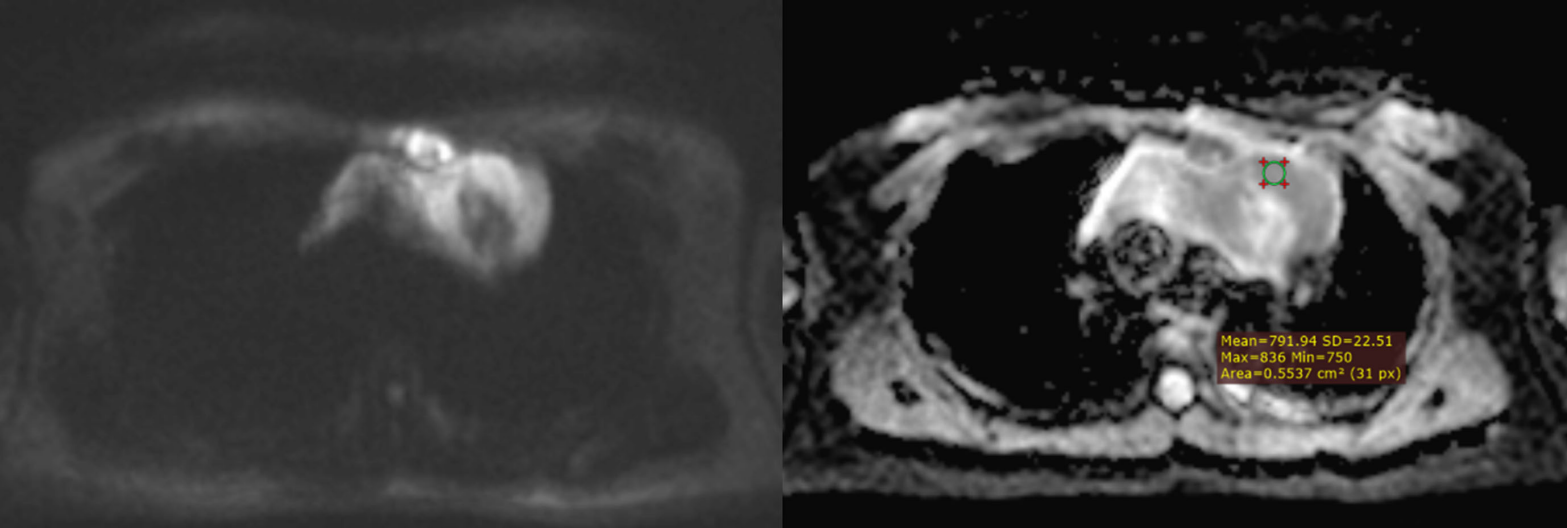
Mediastinal diffuse large B-cell lymphoma. A mediastinal diffuse large B-cell lymphoma having areas of restricted diffusion, which were hyperintense on DWI (b = 2000 sec/mm2) and correspondingly hypointense on ADC map. The mean of 3 ADC values obtained by using the hot-spot-ROI-based approach was 0.75 x 10-3 mm2/sec.
